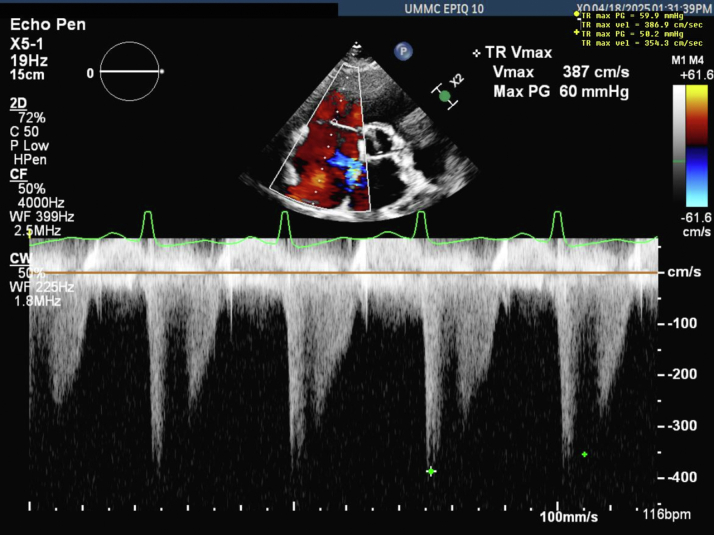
Transthoracic Echocardiography at 27 Weeks Gestation Patient was euthyroid at 27 weeks gestation, one week prior to delivery. Tricuspid valve regurgitation with a TR max velocity of 411.4 cm/s, correlating with an RVSP of 67 mm Hg. There is an increase in estimated RVSP because of physiological changes during pregnancy despite improvement in hyperthyroidism. RVSP = right ventricular systolic pressure; other abbreviations as in Figure 2.
